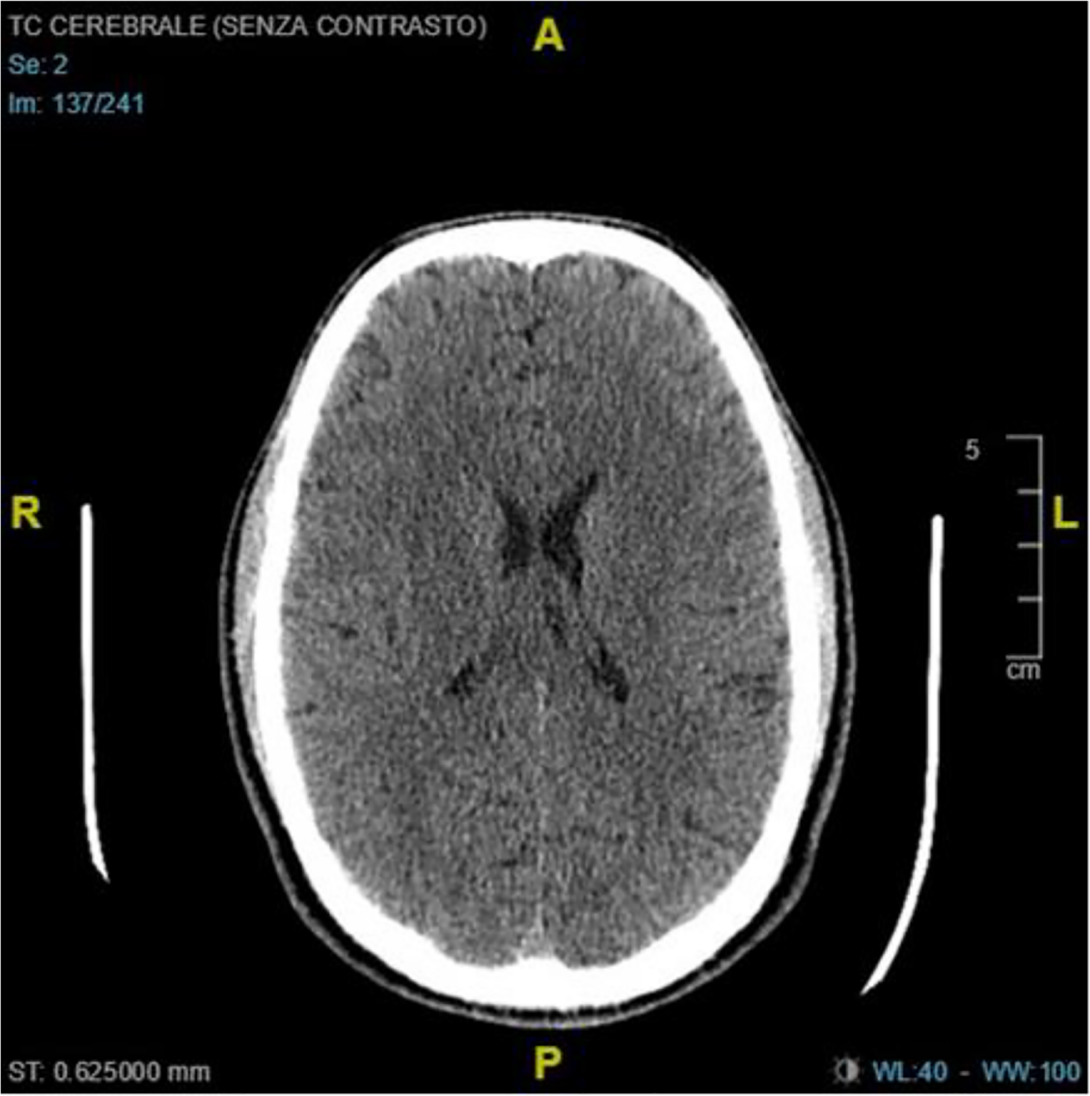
Normal brain CT scan at the entrance
